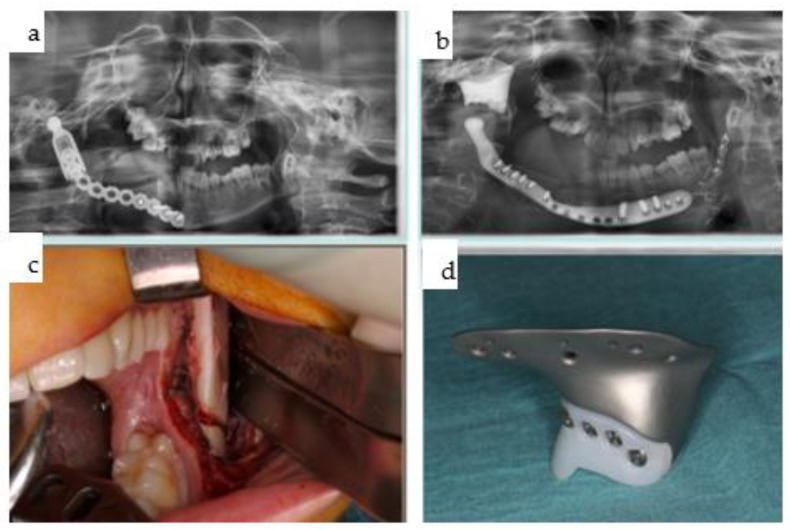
(a–d): Two orthopantomograms show the transition from the non-patient-specific 2.4 reconstruction plate with a condylar attachment without a fossa component (DePuy Synthes^®^, Raynham, MA, USA) (a) to a right patient-specific total joint replacement (Zimmer Biomet [Warsaw, Indiana, USA]) with a long boom serving as a contralateral mandibular implant extension for rigid fixation (b). Initially, bone grafting for the lateral right mandible was performed to facilitate a conventional dental implant rehabilitation protocol, which was later altered. The intraoral view (c) depicts a compensatory contralateral sagittal split osteotomy, while the hybrid metallic and polyethylene cranial and fossa components were documented intraoperatively prior to insertion (d).
